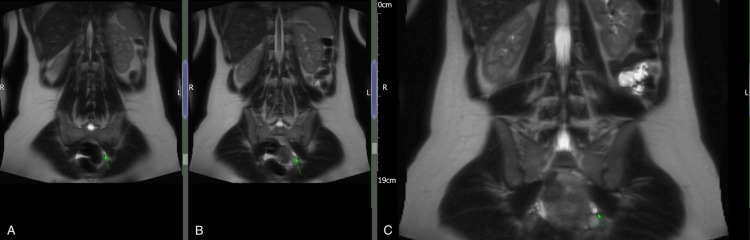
(A-C) Coronal T2-weighted MRI sequences showing a tubular structure in the left adnexa, raising suspicion for a haematosalpinx.
